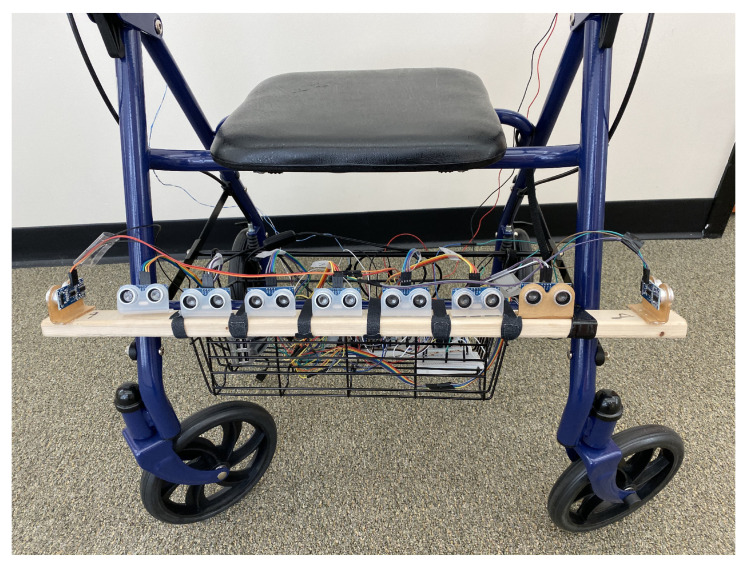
The rollator configured with nine HC-SR04 ultrasonic sensors. Seven sensors are facing forward to capture obstacles in a wide area in front of the walker. To facilitate navigational guidance, two sensors (one on the left and the other on the right) are capturing obstacles.
